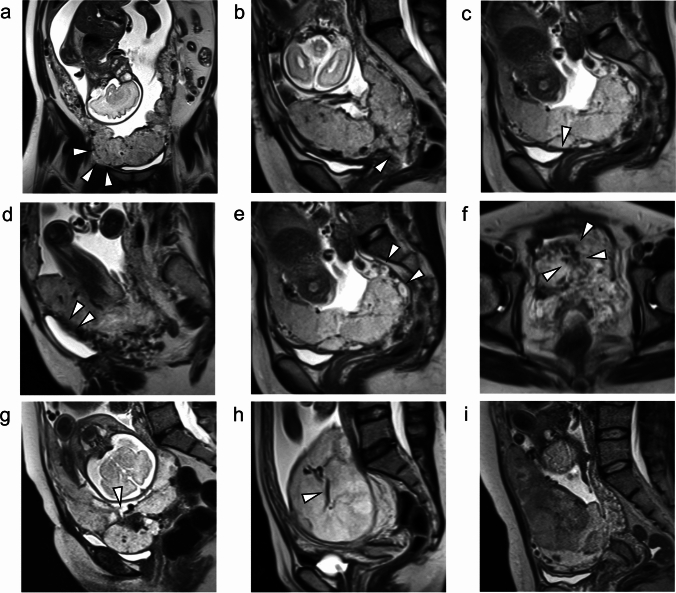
Representative MRI (T2WI) features of PAS. a Placental/Uterine bulging (arrowheads). b Placental protrusion into the internal os (arrowhead). c Myometrial thinning or focal disruption (arrowhead). d Loss ofT2 hypointense interface (arrowheads). e Myometrial hypervascularity (arrowheads). f Serosal hypervascularity (arrowheads). g Placental ischemic infarction/recess and intraplacental T2 dark bands (arrowhead). h Abnormal intraplacental vascularity (arrowhead). i Placental heterogeneity. All images shown were acquired with single-shot turbo spin-echo (HASTE) sequence
